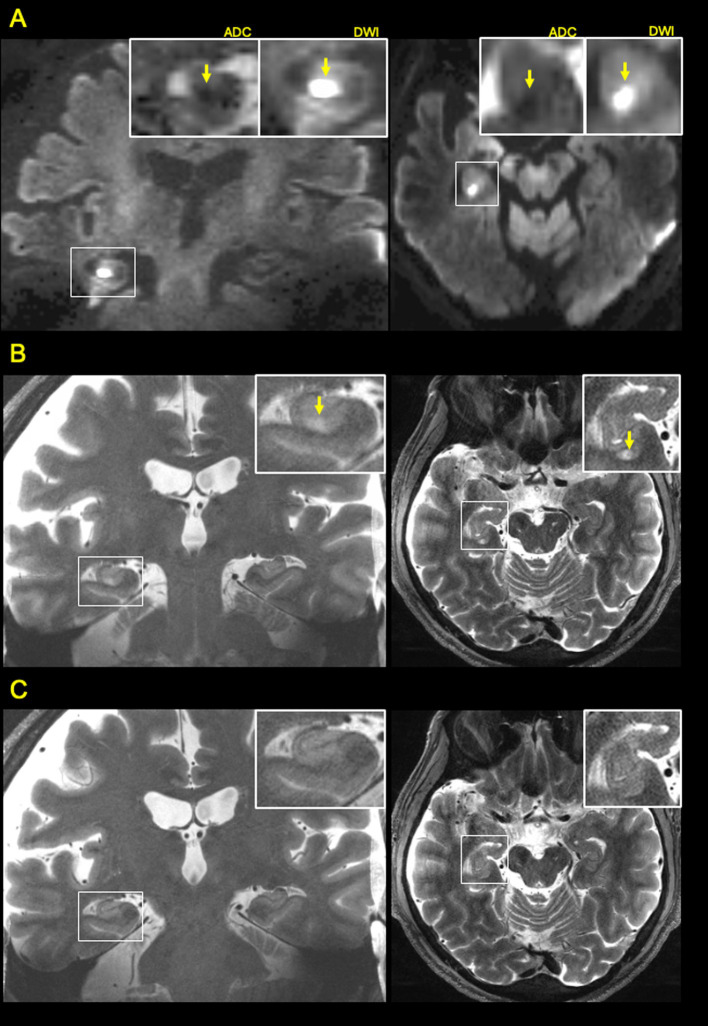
Serial 7 T MRI findings in Case 4. (A) Acute 7 T diffusion-weighted imaging (DWI) obtained 35 h after symptom onset demonstrates a focal CA1 hyperintensity (arrow). Corresponding apparent diffusion coefficient (ADC) maps show focal hypointensity at the site of the DWI lesion, confirming restricted diffusion. Magnified views of the lesion are shown in the upper-right corner of each image; the left inset displays the ADC map, and the right inset displays the DWI image. (B) Simultaneous 7 T T2-weighted imaging shows a corresponding cortical-layer signal abnormality in CA1 (arrow), evident on both coronal and axial views. (C) Follow-up 7 T T2-weighted imaging performed on day 15 demonstrates complete resolution of the previously observed CA1 abnormality.
